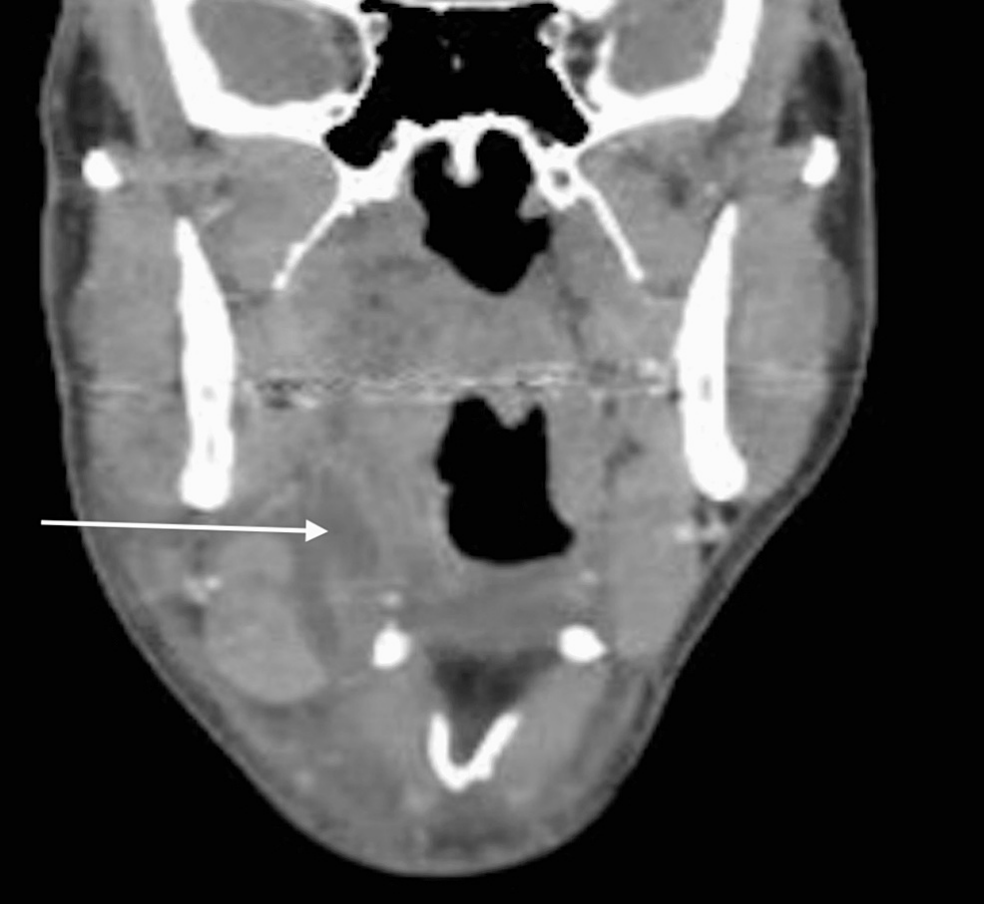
Coronal view of CT scan at initial presentation White arrows represent fluid collection surrounding the right submandibular gland.
